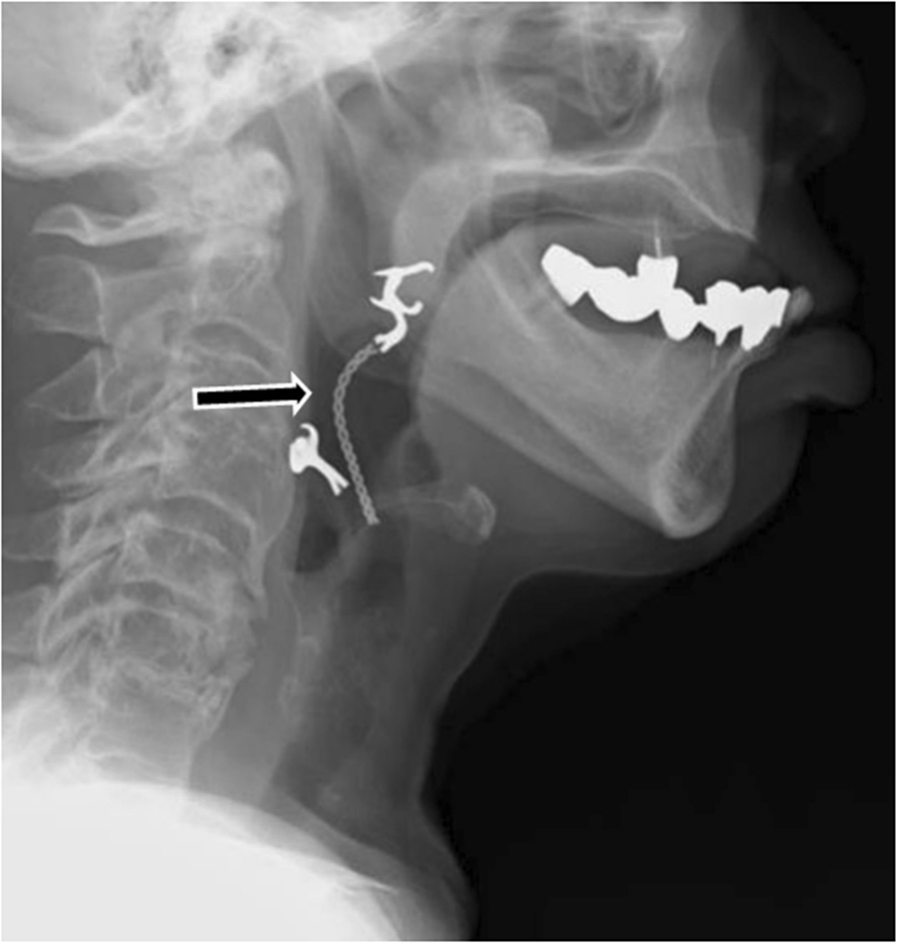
Radiograph of the neck showing a denture lodged in the pharygo-larynx (arrow).
